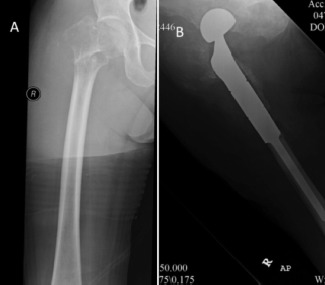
(A) Radiograph of a patient with metastatic disease of the proximal femur involving the intertrochanteric region. (B) Postoperative radiograph.
